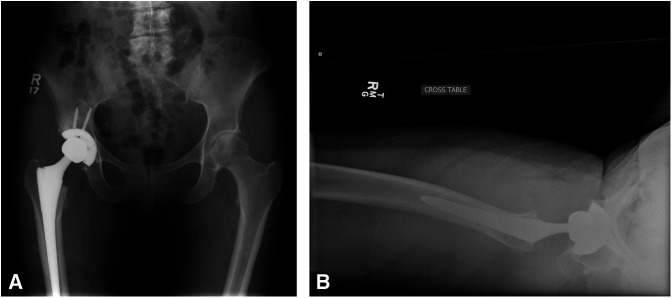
A and B, AP radiograph of the pelvis and lateral radiograph of the right hip.
